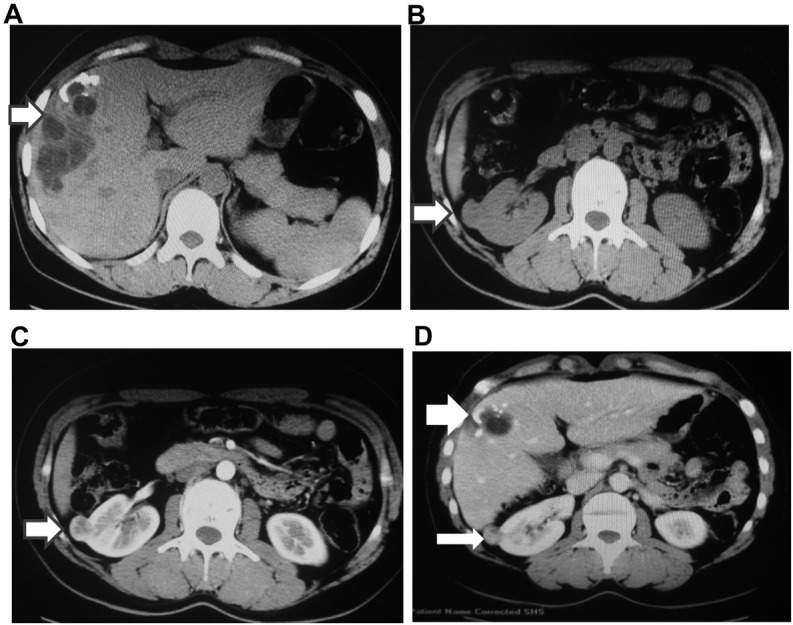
Photographs of computed tomography (CT) of the liver and kidney. A. CT-scan (plain) showing the parasitic cystic lesion in the right hepatic lobe (indicated by arrow). B. CT-scan (plain) showing the tumor in the right kidney (indicated by arrow). C. The lesion of kidney was significantly enhanced after contrast enhancement (indicated by arrow). D. CT-scan showing carcinoma of kidney (thin arrow) complicated with echinococcal cyst of the liver (thick arrow).
